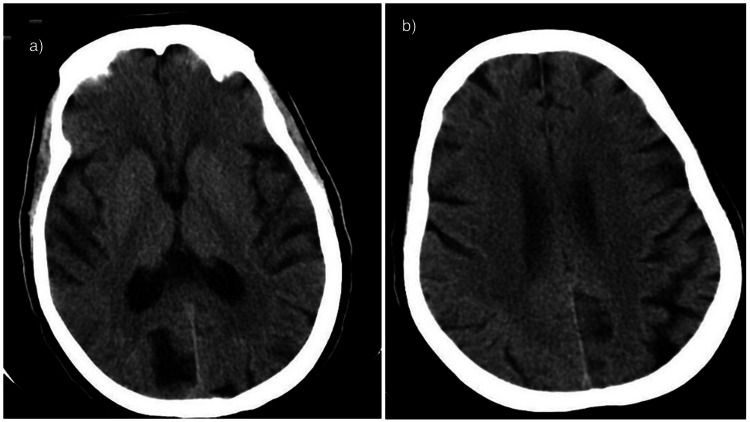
A and B showing the computed tomography (CT) of the brain with a good aspect score
